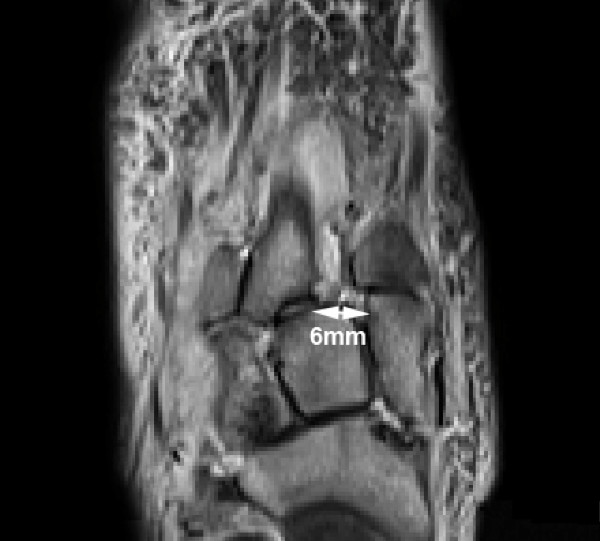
STIR sequence oblique axial view of the right foot. This shows the 6 mm lateral displacement of the 2nd and 3rd metatarsals and a completely ruptured Lisfranc ligament which should usually be seen crossing the 1^st ^metatarsal space obliquely. The ruptured ligament would explain why the typical "fleck sign" was absent on the plain radiograph as a ruptured ligament will not avulse the lateral border of the medial cuneiform or the medial border of the 2^nd ^metatarsal base.
